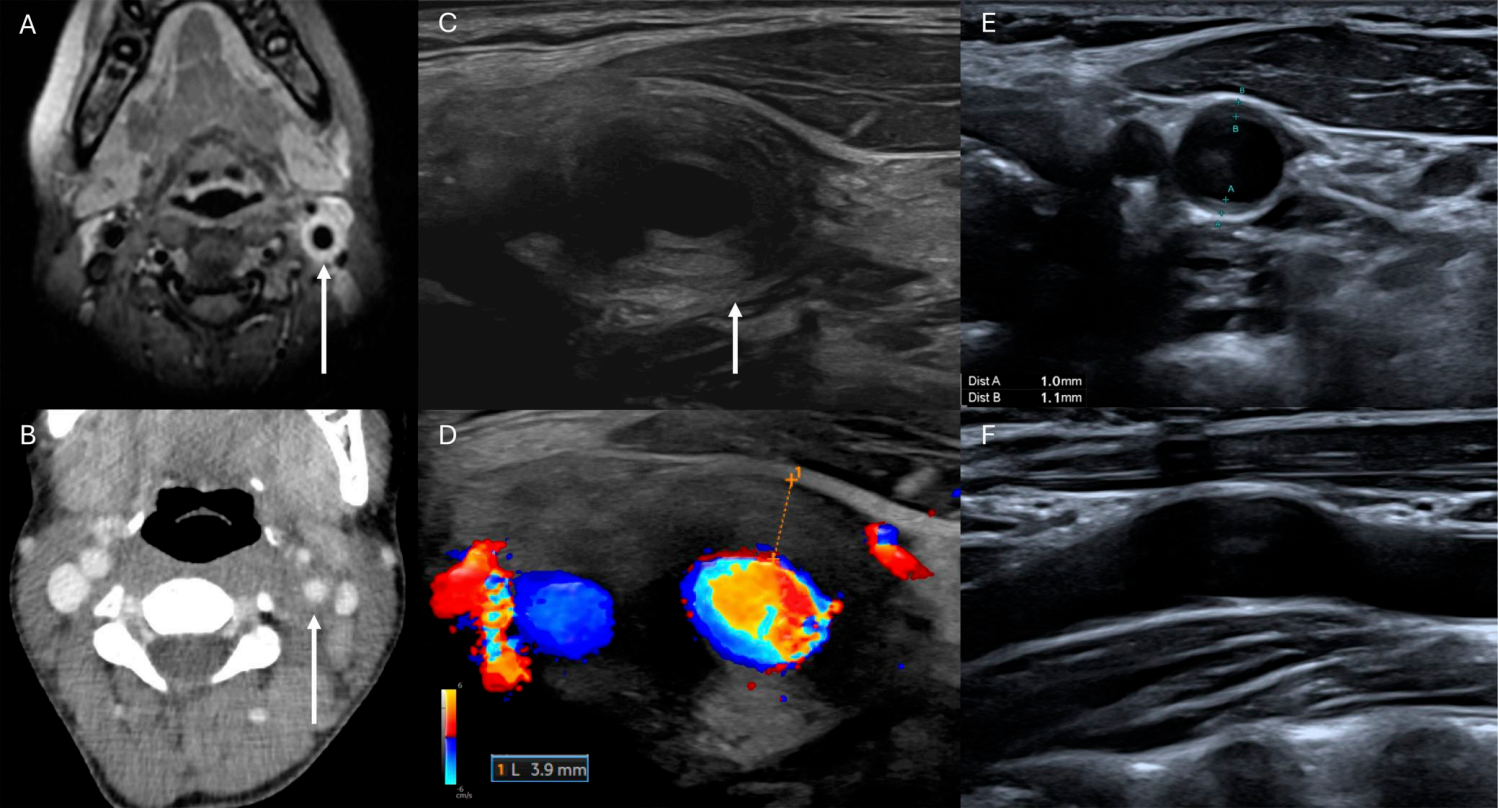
Imaging of perivascular inflammation of the left internal carotid artery at diagnosis and at 4 wk of treatment. (A–F) At diagnosis: circumferential parietal thickening (white arrows) of the left carotid artery wall on axial cervical views of MRI (A), CT scan (B), US (C), and Doppler (D). At midterm treatment: regression of wall thickening on axial (E) and sagittal (F) US views.
